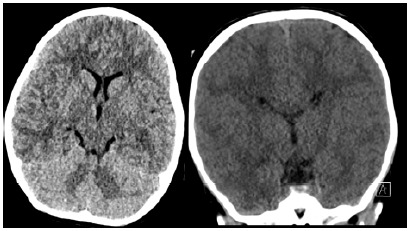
Representative axial and coronal images from a computed tomography head of a 19-month-old girl with seizure.
